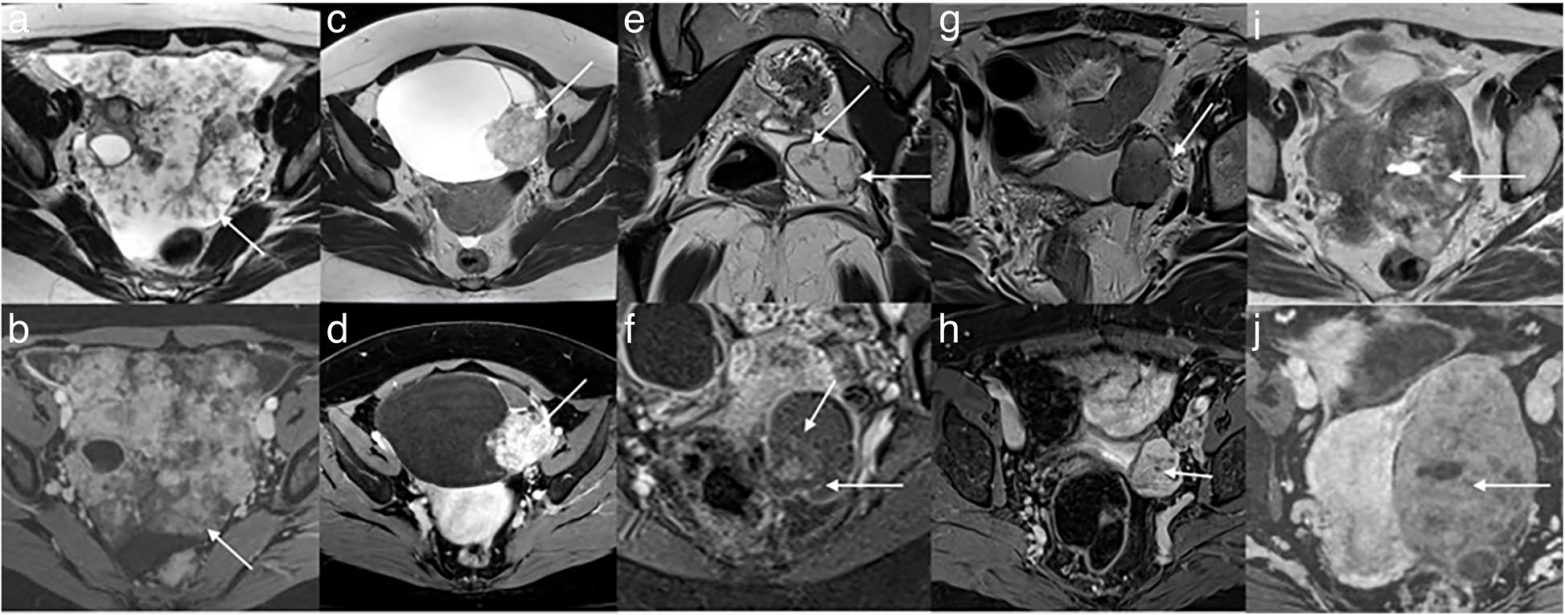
Example of solid tissue descriptors: a Axial T2-weighted sequence without fat saturation. b Axial unenhanced T1-weighted Water sequence showing papillary projections in bilateral serous borderline ovarian lesions (arrows). c Axial T2-weighted sequence without fat saturation. d Axial unenhanced T1-weighted Water sequence showing a large mural nodule (arrows) in a high-grade serous ovarian cancer. e Axial T2-weighted sequence without fat saturation. f Axial unenhanced T1-weighted Water sequence showing irregular septations (arrows) in bilateral serous borderline ovarian lesions. g Axial T2-weighted sequence without fat saturation. h Axial unenhanced T1-weighted Water sequence showing irregular wall (arrows) in a serous borderline bilateral ovarian tumor. i Axial T2-weighted sequence without fat saturation. j Axial unenhanced T1-weighted Water sequence showing irregular septations showing large solid component (arrows) high-grade serous ovarian cancer
